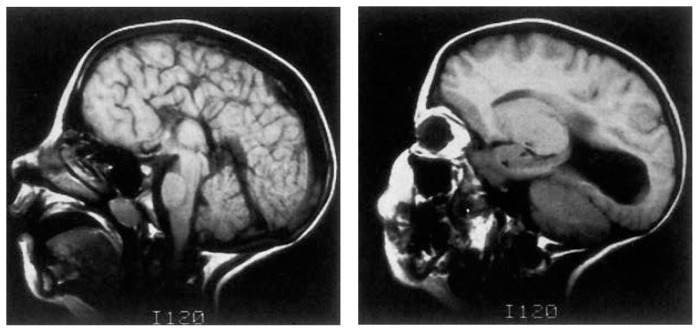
Two magnetic resonance images from a child with fetal alcohol syndrome demonstrating agenesis of the corpus callosum (left) and colpocephaly (right), a feature often associated with callosal agenesis.
